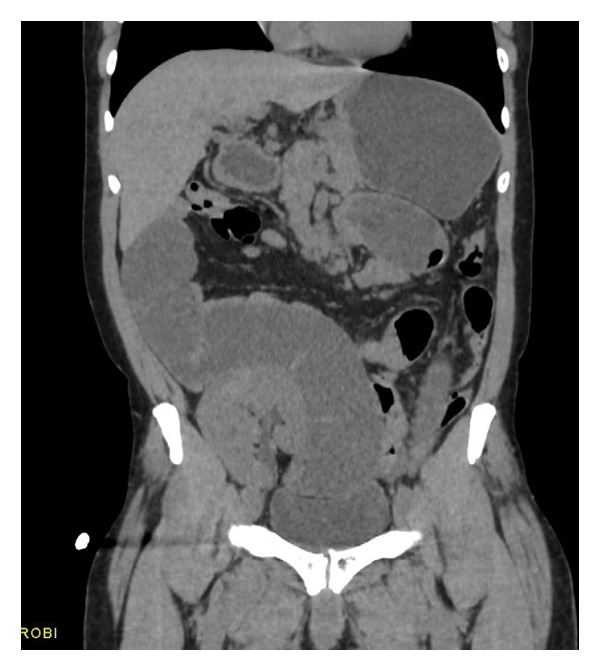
Unenhanced CT of the abdomen: coronal image at the level of the transplant kidney demonstrating dilated fluid‐filled loops of small bowel measuring up to 4.4 cm in diameter.
